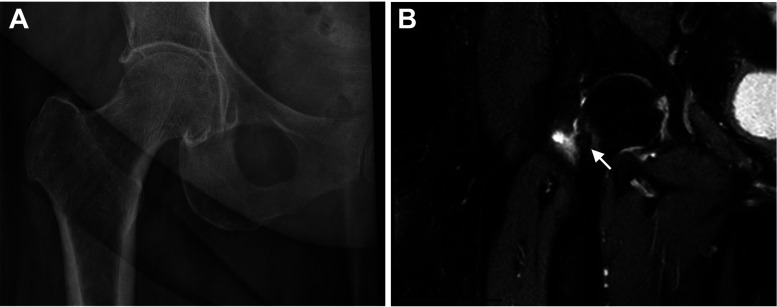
Representative radiological images. (A) An X-ray of the right hip shows moderate hip joint narrowing and degenerative joint disease, with no distinct lesion identified. (B) Coronal T2-weighted image of the right femur shows a 6.4mm round focus of intermediate signal intensity (arrow) contiguous with the cortical margin, along with surrounding bone marrow edema.
